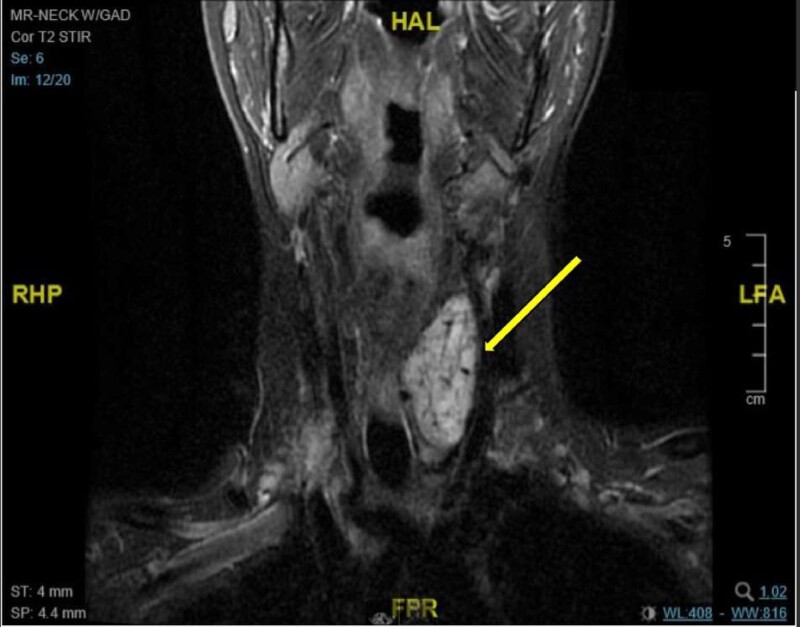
MRI scan of the neck with gadolinium (coronal MRI) demonstrating a mass in the left infrahyoid neck that is 5.6 cm in the maximal craniocaudal dimension, 2.8 cm in the anteroposterior dimension, and 2.4 cm in the mediolateral dimension. The mass is isointense to muscle on the T1-weighted sequence and demonstrates moderate T2 hyperintensity with avid postcontrast enhancement in keeping with a hypervascular mass with arterial supply that arose from the thyroid inferior mesenteric artery and left inferior thyroid artery.
